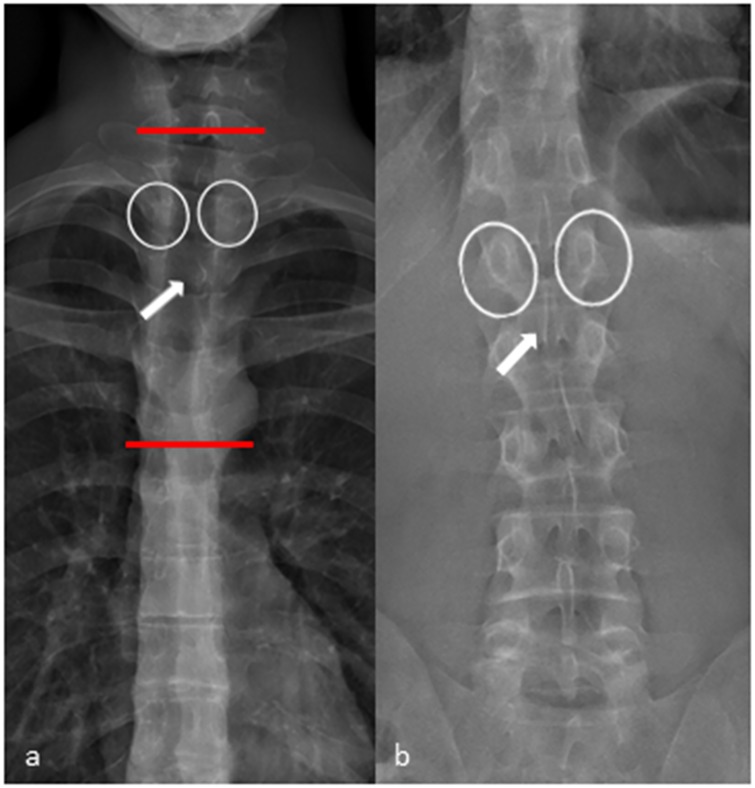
Frontal view X-ray performed with EOS. (a) Frontal view of the thoracic spine. The pedicles (white circles) and the shape of the processus spinosus (white arrow) are difficult to identify in the upper and middle thoracic spine (area in between the red lines). (b) In the lower thoracic spine and in the lumbar spine identifying the pedicles (white circles) and the shape of the proccessus spinosus (white arrow) is straight forward.
